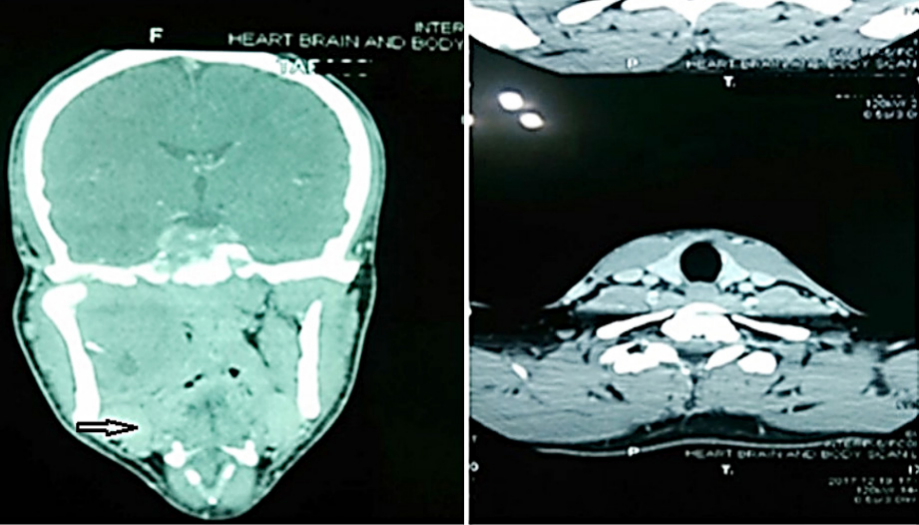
CT scan of face and neck is showing hypodense mass (→). Erosive changes are evident in lateral wall of the right orbit (B).
